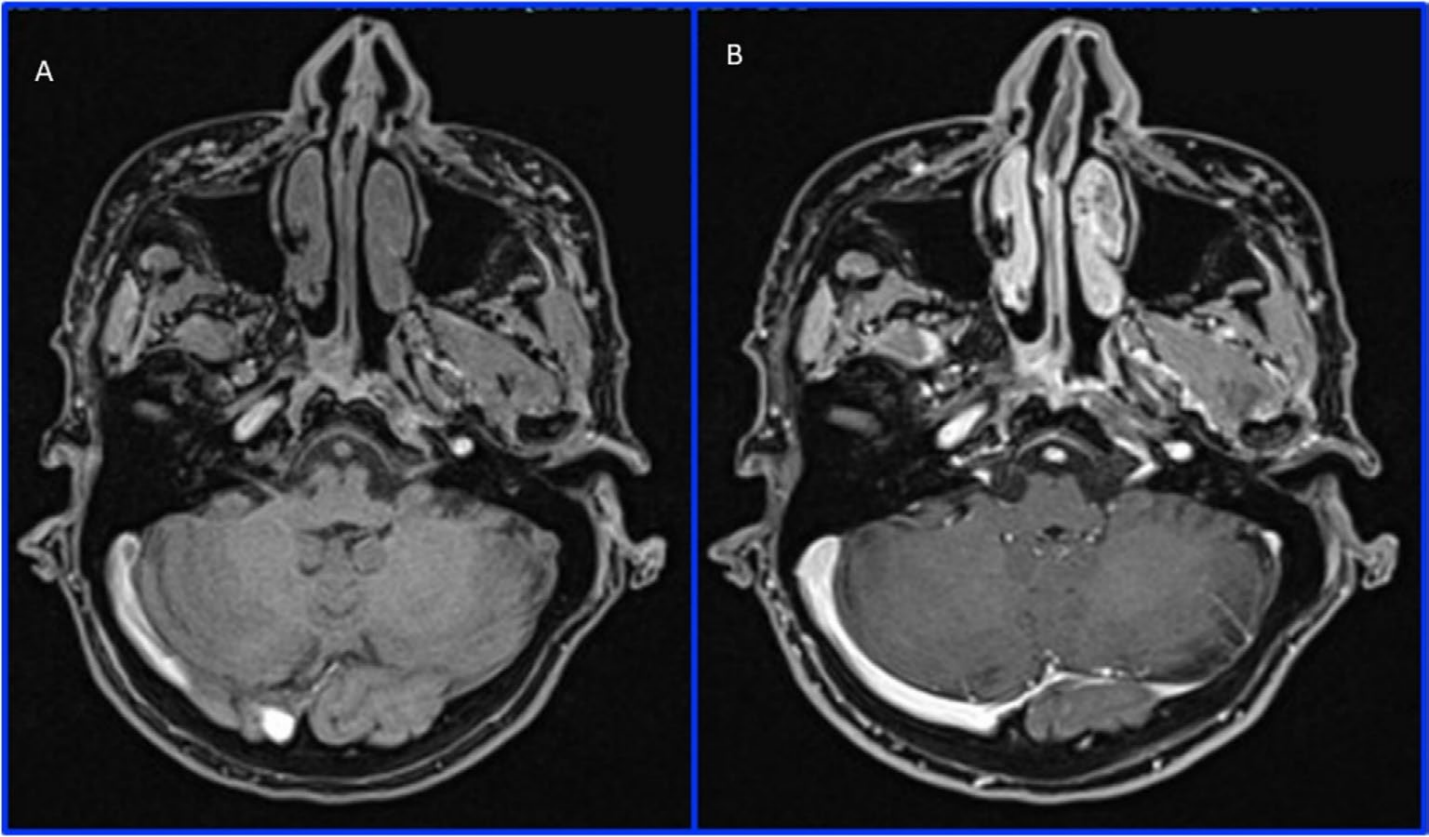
MRI: Axial T1 VIBE FAT SAT (A) e T1 VIBE FAT SAT after contrast medium administration (B) of the III MRI, showing no difference between with or without contrast images in highlighting the HA filler, which appears as low signal areas. We could not see a significant difference between the first and the second MRI (performed more than 6 months later), and in the third MRI, the filler was also well represented.
